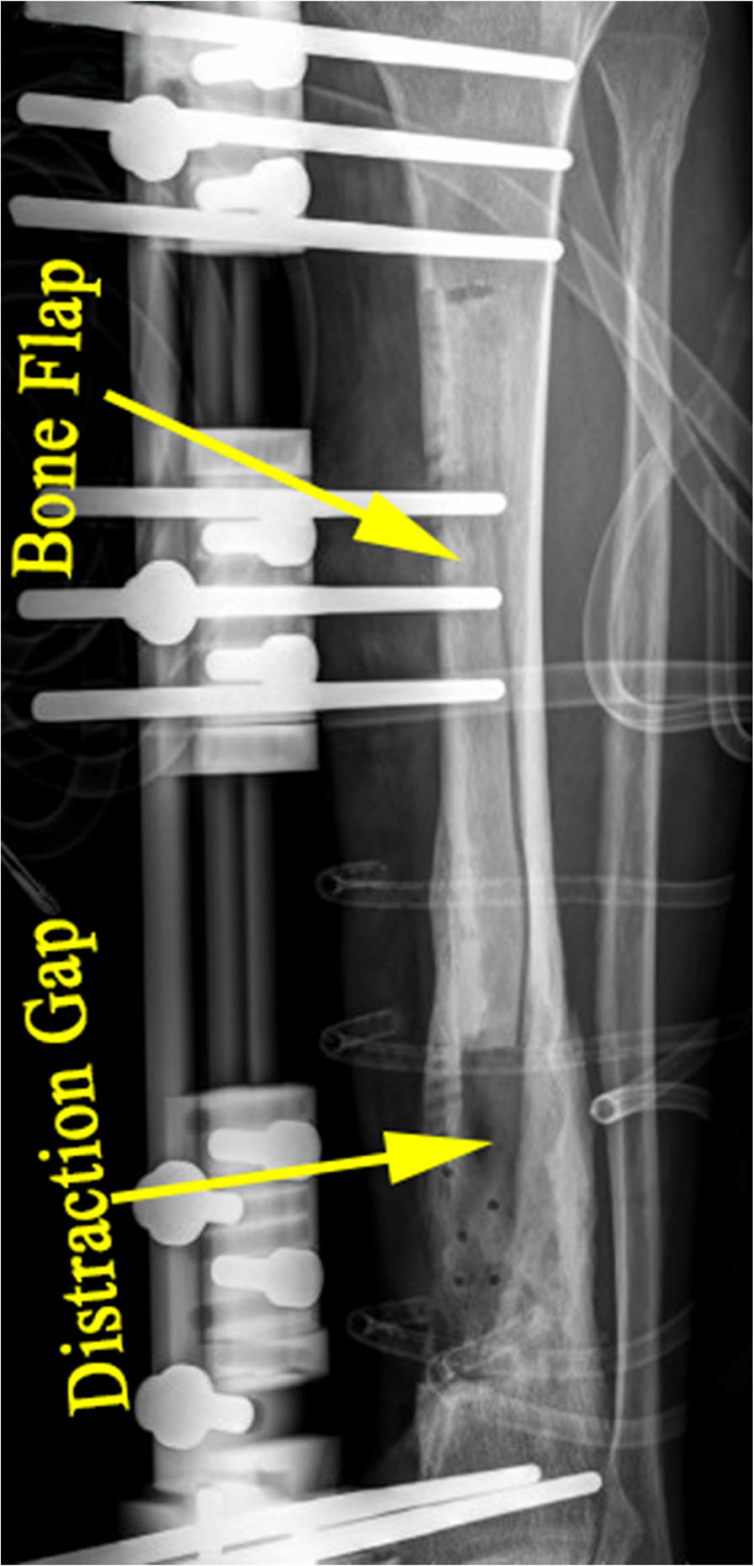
Postoperative lateral radiograph of the patient treated with monolateral external fixator
